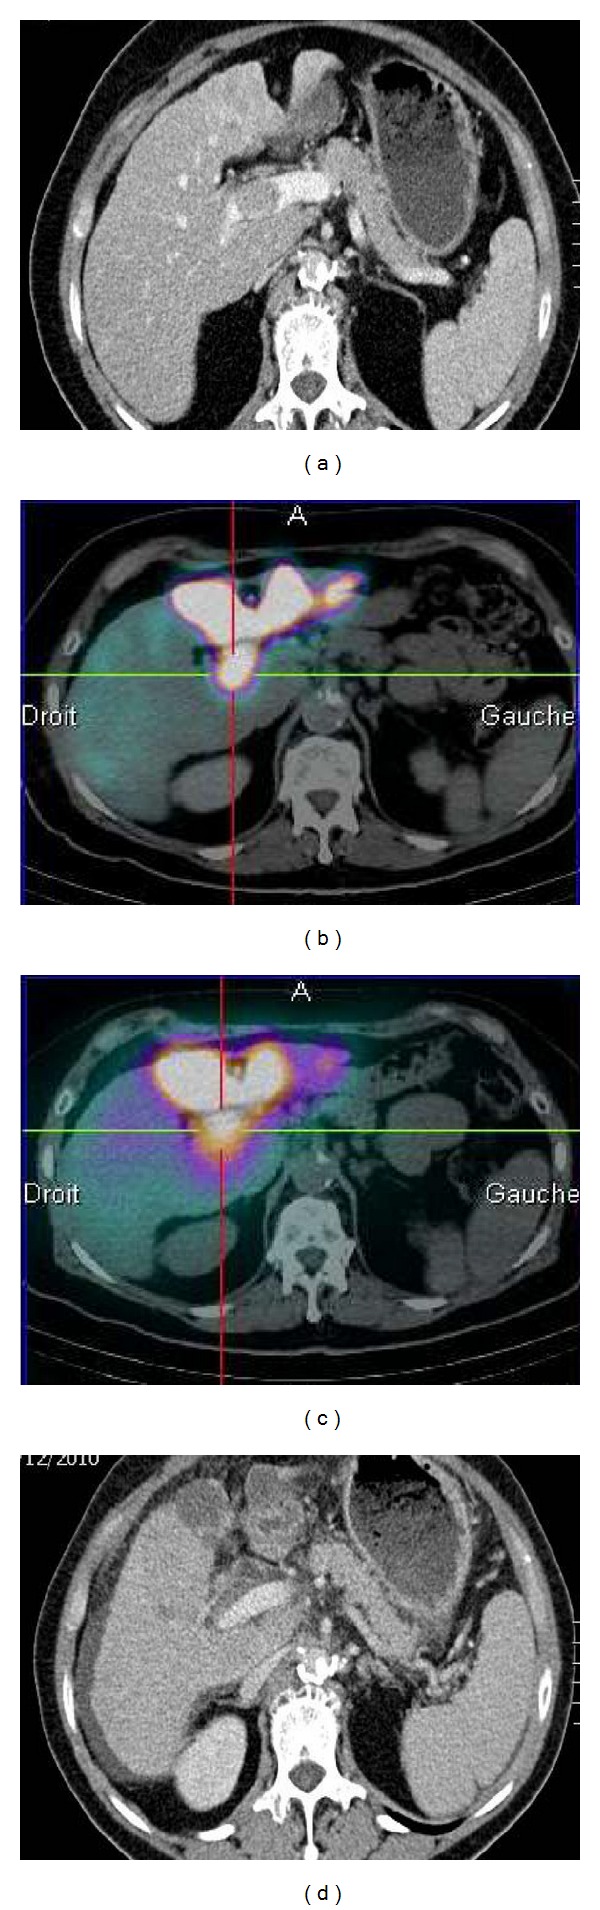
MAA SPECT/CT and CT scans showing implantation of microspheres in an PVTT and the involution of this PVTT after radioembolization: (a) Baseline CT scan, (b) MAA SPECT/CT showing a high MAA uptake in the PVTT, (c) Posttherapeutic Bremsstrahlung SPECT/CT after the injection of 4.5 GBq of 90Y-loaded glass microspheres: high uptake in the PVTT confirming the accurate implantation of microspheres in the PVTT, and (d) CT scan at 13 months.
